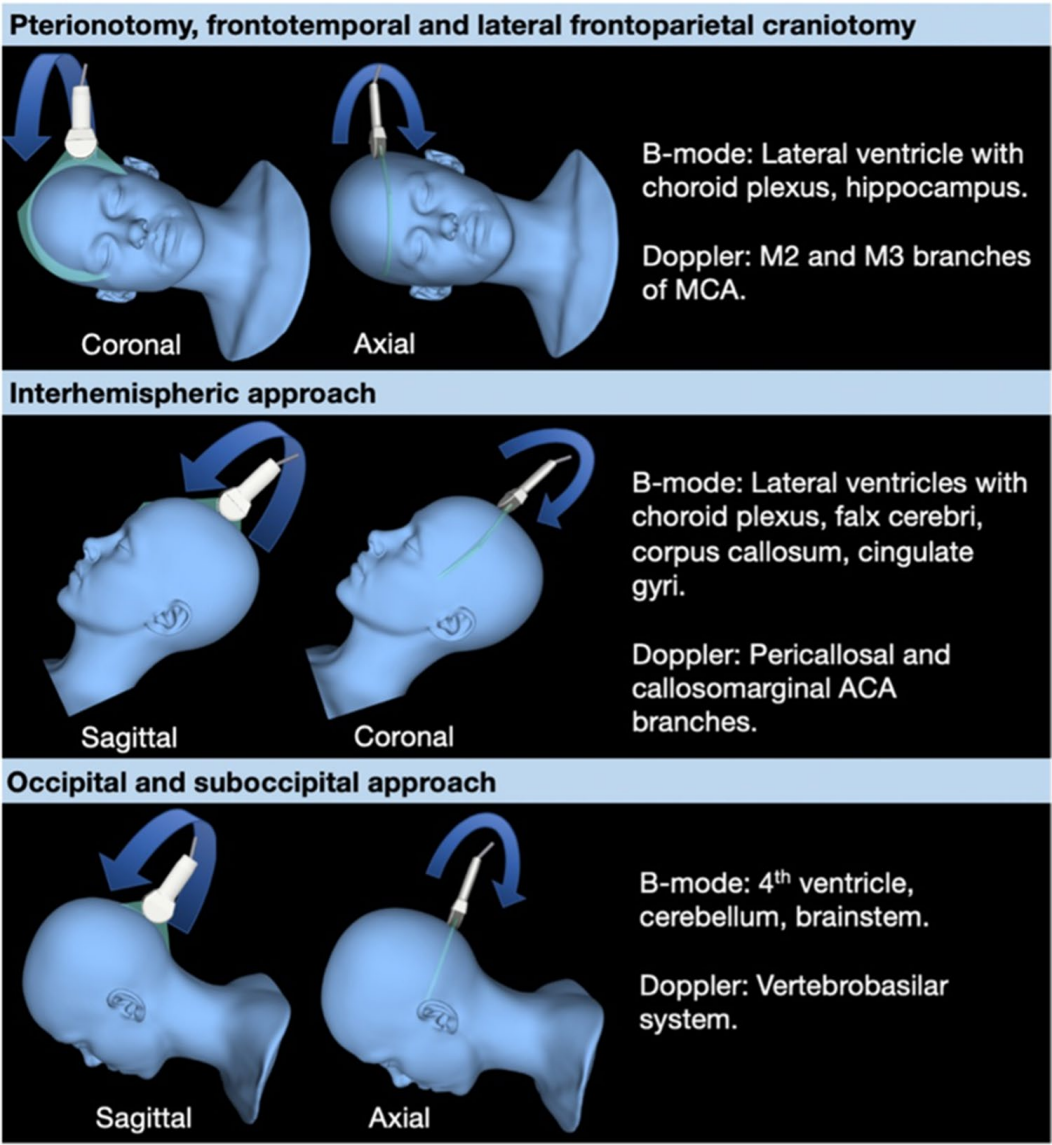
Recommended orthogonal ultrasound fans for different craniotomies with expected anatomical and vascular landmarks. Model of orthogonal ultrasound sweeps for common craniotomy sites. Probe positioned to achieve views that approximate to standard anatomical planes on CT/MRI. Patient positioned to ensure the craniotomy is as horizontal as possible to allow retention of fluid in the resection cavity for optimal ultrasound coupling
